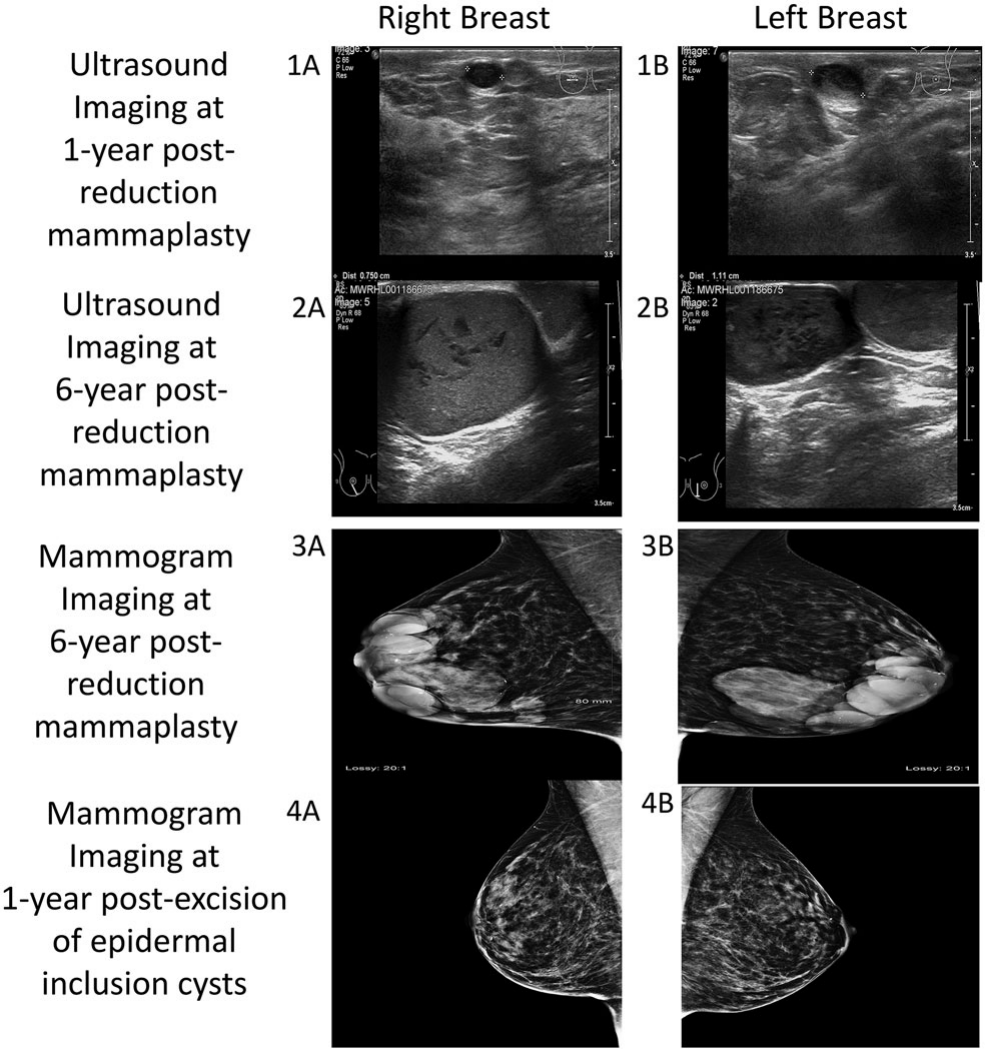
Imaging series showing progression of bilateral epidermal inclusion cysts of the breast. Ultrasound imaging: at one-year post-reduction mammaplasty (1A,1B) and at six-year post-reduction mammaplasty (2A,2B). Mammogram imaging at six-year post-reduction mammaplasty (3A,3B) and at one-year post-excision of epidermal inclusion cysts (4A,4B).
